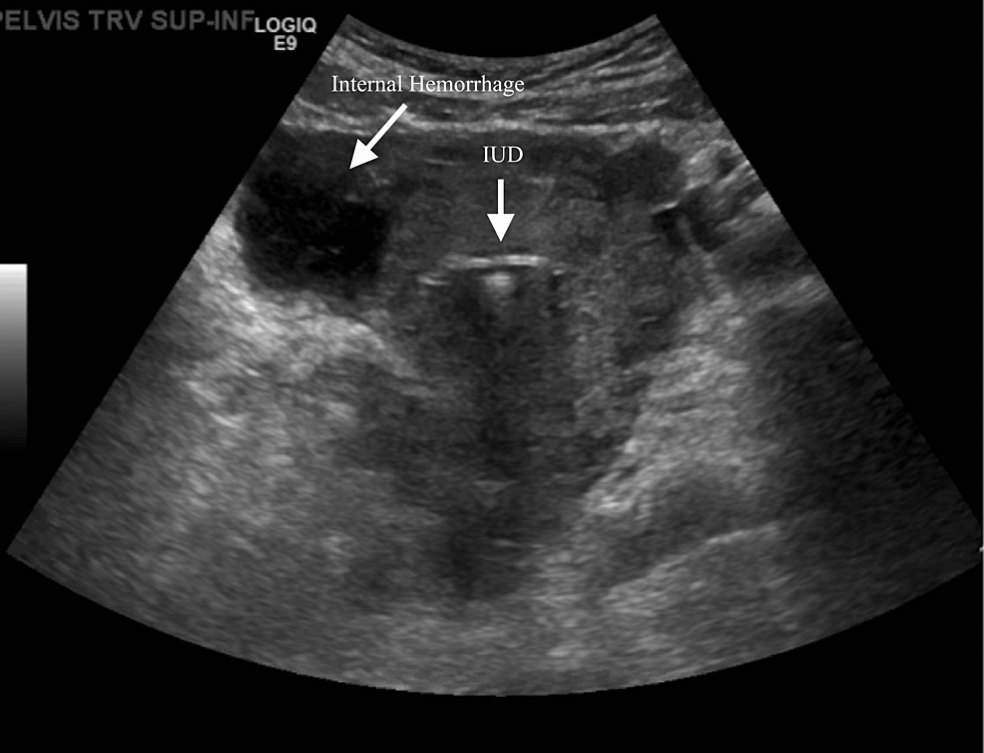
Ultrasound of the IUD in proper position with evidence of internal hemorrhage. IUD = intrauterine device
